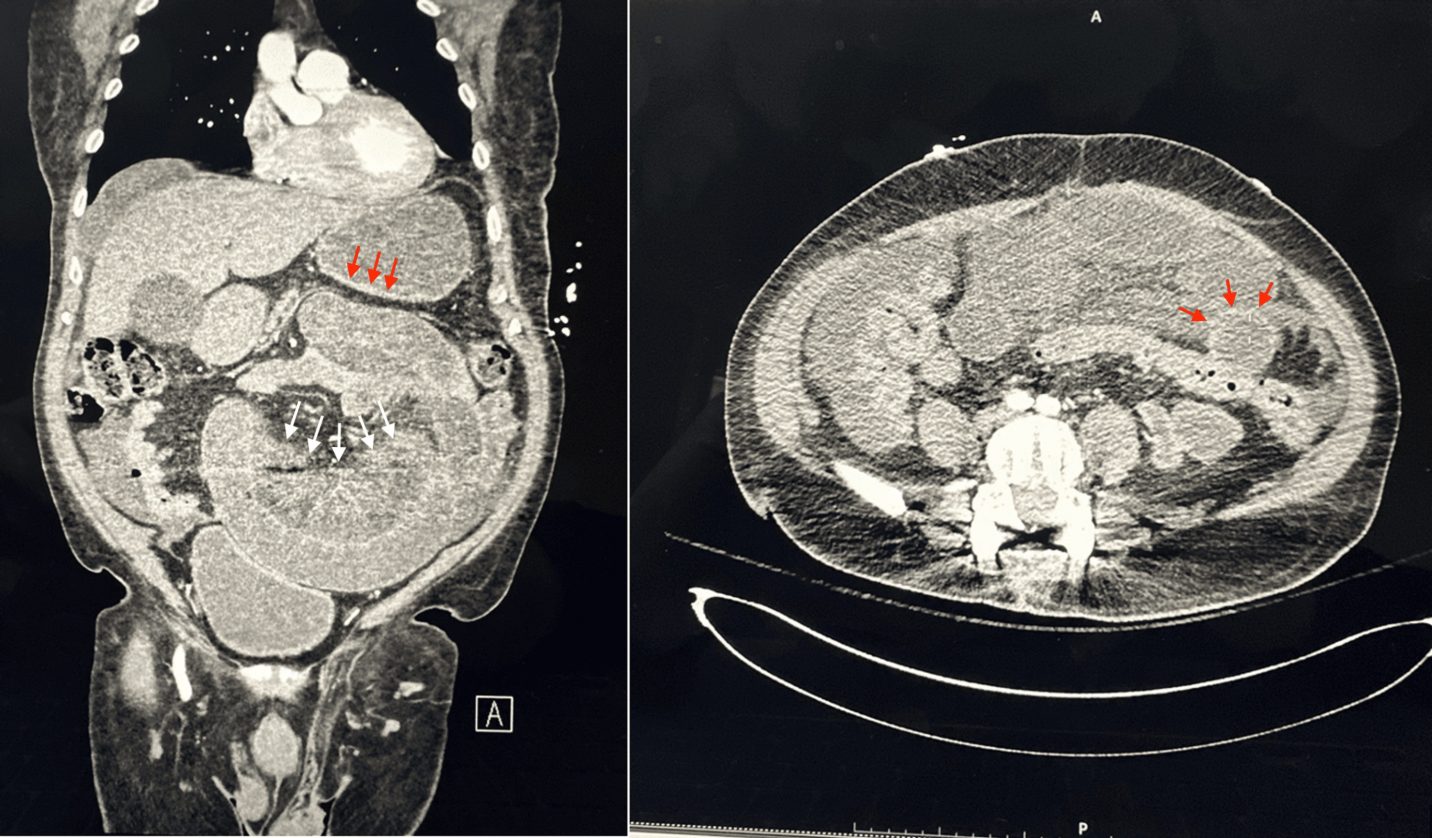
Small bowel obstruction A CT scan of the abdomen and pelvis demonstrates dilated air-filled small bowel loops with distal decompression consistent with typical findings of complete small bowel obstruction, along with thickened and increased attenuation of the bowel wall (red arrows) and mesenteric edema (white arrows) associated with intestinal ischemia.
